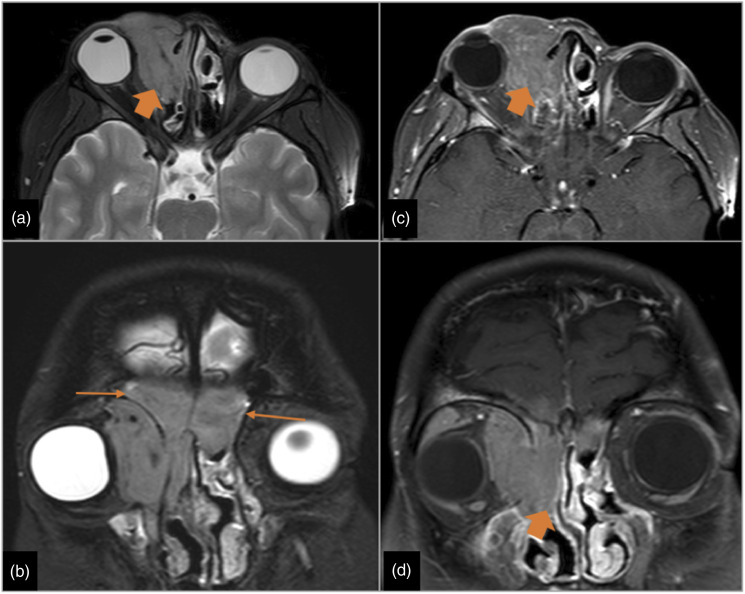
B-cell lymphoproliferative disorder. A 21-year-old woman with double-LT due to cystic fibrosis, started with right exophthalmos 5 months after the transplantation. Figures: axial MRI T2-weighted sequence (a), coronal MRI Stir sequence (b), axial MRI intravenous contrast T1-weighted sequence (c) and coronal MRI intravenous contrast T1-weighted sequence (d). A right orbital mass that displaces the eyeball (thick arrow in a and b) and reaches the right nasal cavity (thick arrow in c) and both frontal sinuses (thin arrows in d). The mass appears hyperintense and intermediate intensity on T2 sequences, hypointense on T1 sequences and shows moderate and uniform post contrast enhancement.
